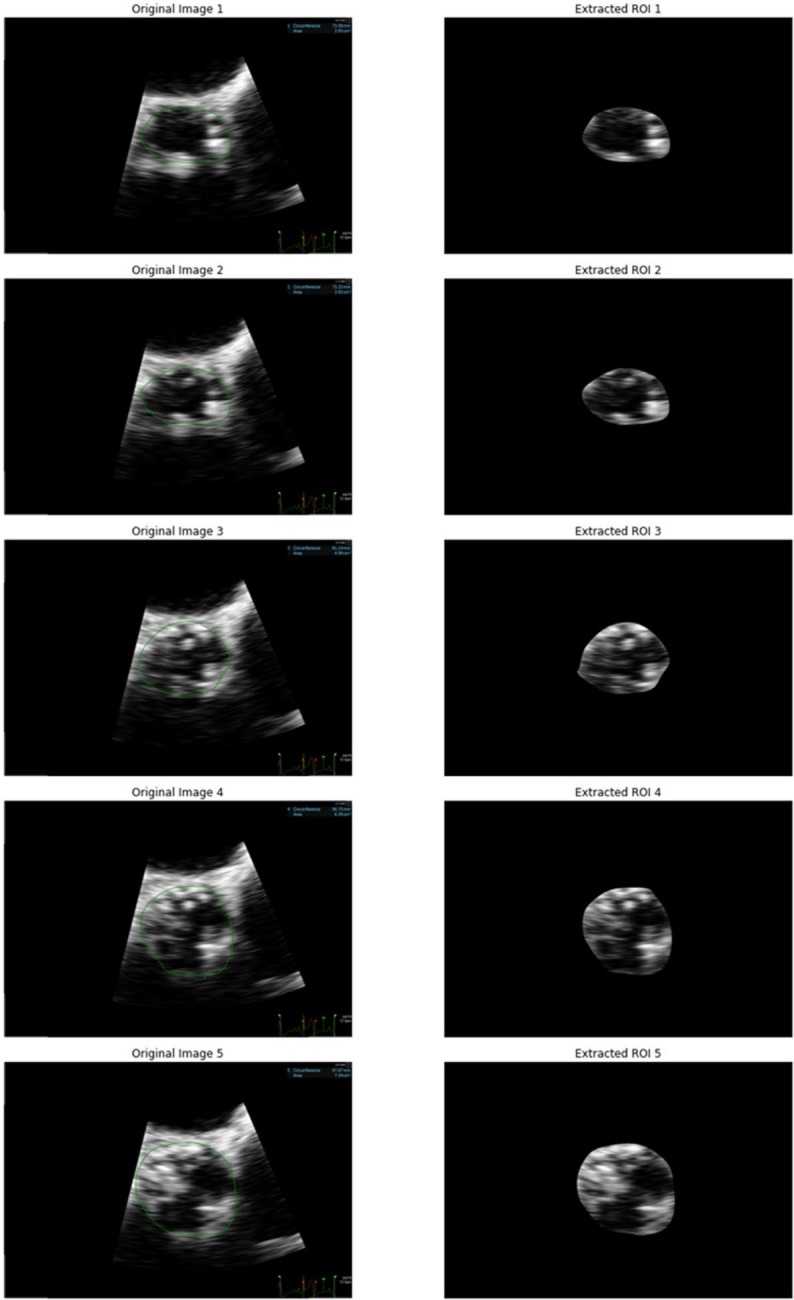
Original echocardiographic images and extracted region of interest (ROI)
